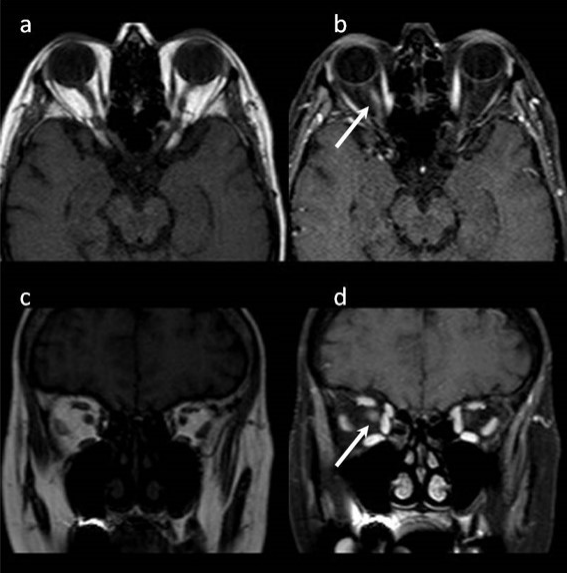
T2 weighted sequence MRI of the brain and orbits revealed high signal intensity in the right optic nerve.
